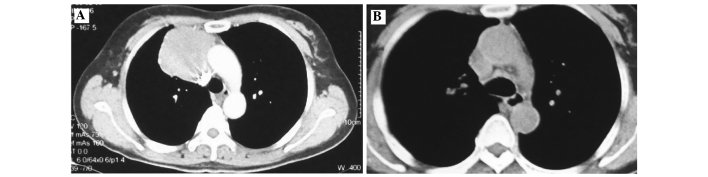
Thoracic computed tomography images (A) prior to and (B) subsequent to surgery.
